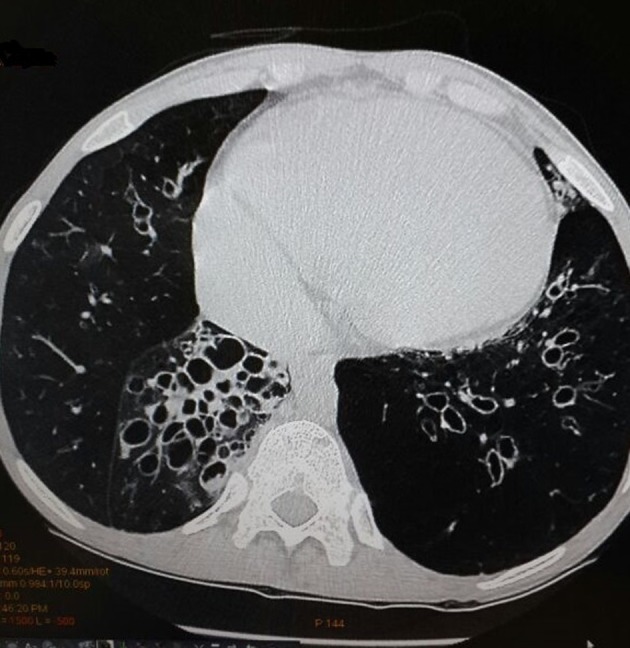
High-resolution computed tomography scan showing cystic bronchiectasis with bronchial wall thickening and mucous plugging.
